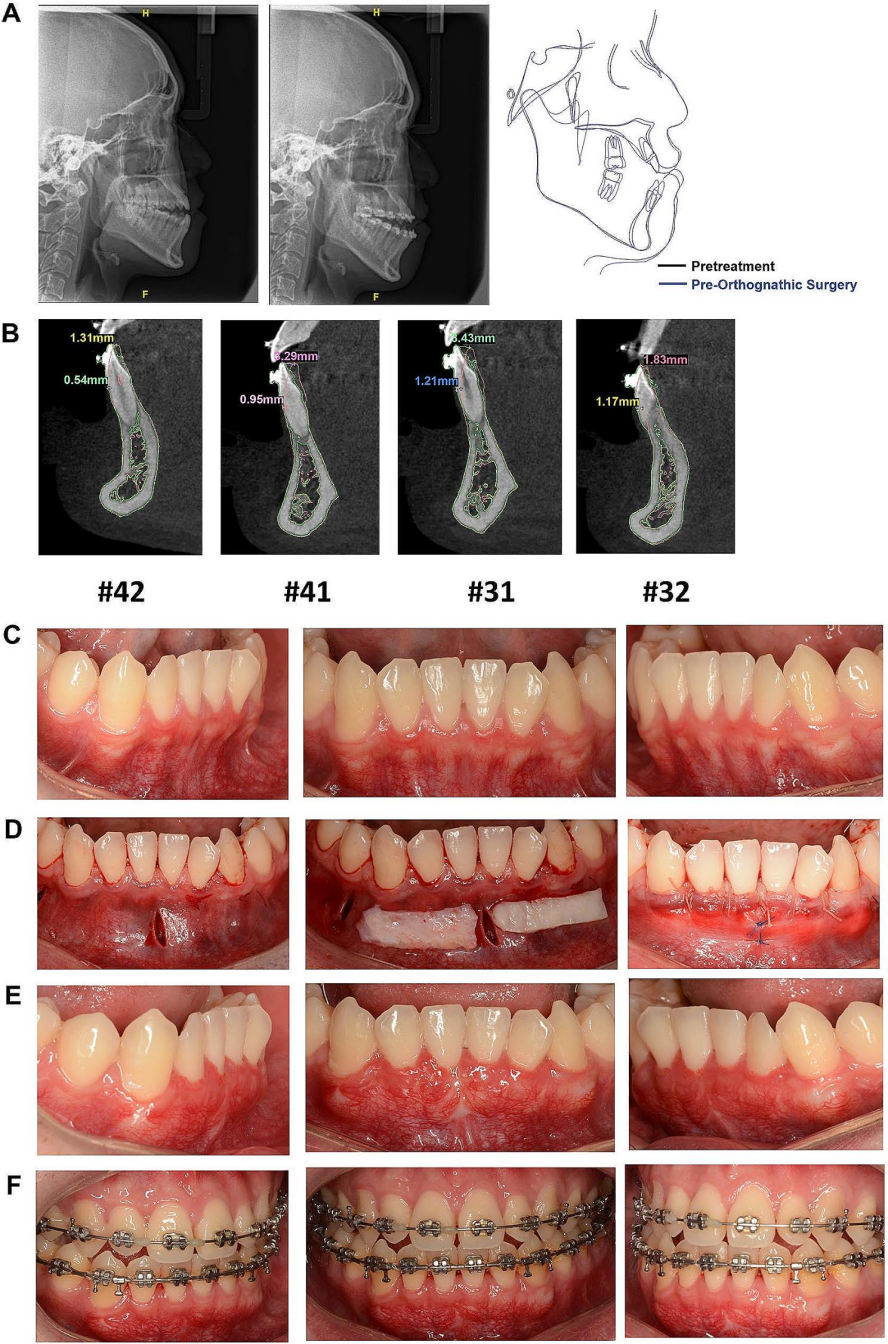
Cephalometric radiographs, CBCT and clinical photographs of case 3. A The comparison of the cephalometric radiographs of the baseline and the end of pre orthognathic surgical orthodontic treatment. B The superimposition images of the #31#32#41#42 CBCT data at baseline and at the end of pre orthognathic surgical orthodontic treatment. C The appearance of the anterior mandibular teeth with thin periodontal phenotype at baseline. D Subperiosteal tunnel was extended from the vestibular to the gingival margin, which accommodated a 2 mm thick and at least 5 mm apico-coronal-dimensioned autogenous connective tissue graft. E The photographs at three months after the PhMT-s surgery F The photographs at the end of orthognathic surgery
